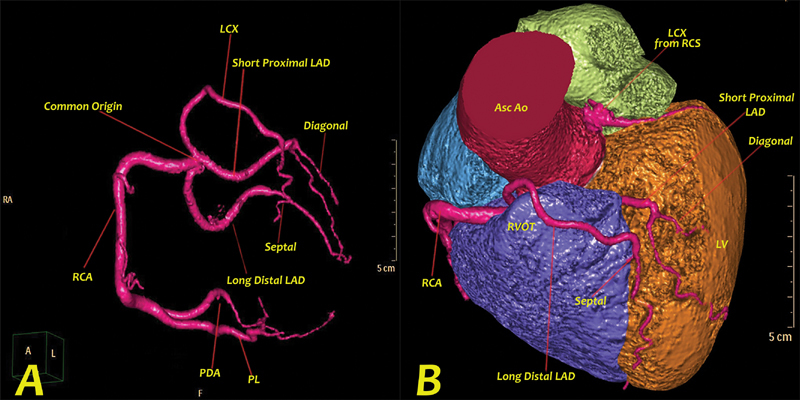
Computed tomography (CT) coronary angiography colored three-dimensional volume rendered CT angiography images showing (
